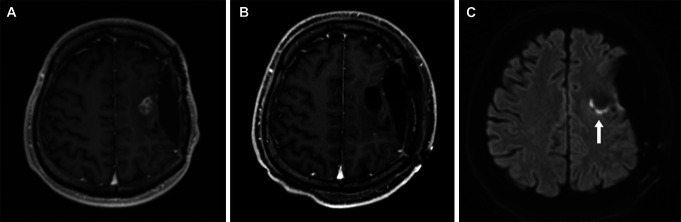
MRI of a 47-year-old man after treated with radiation, chemotherapy, and A, tumor treating fields showing progressive disease of glioblastoma. B, Awake craniotomy to achieve gross total resection is performed. Fourteen hours after surgery, the worsening of right-sided paralysis appeared. C, DWI-MRI showing cerebral ischemia in the deep white matter (arrow).
